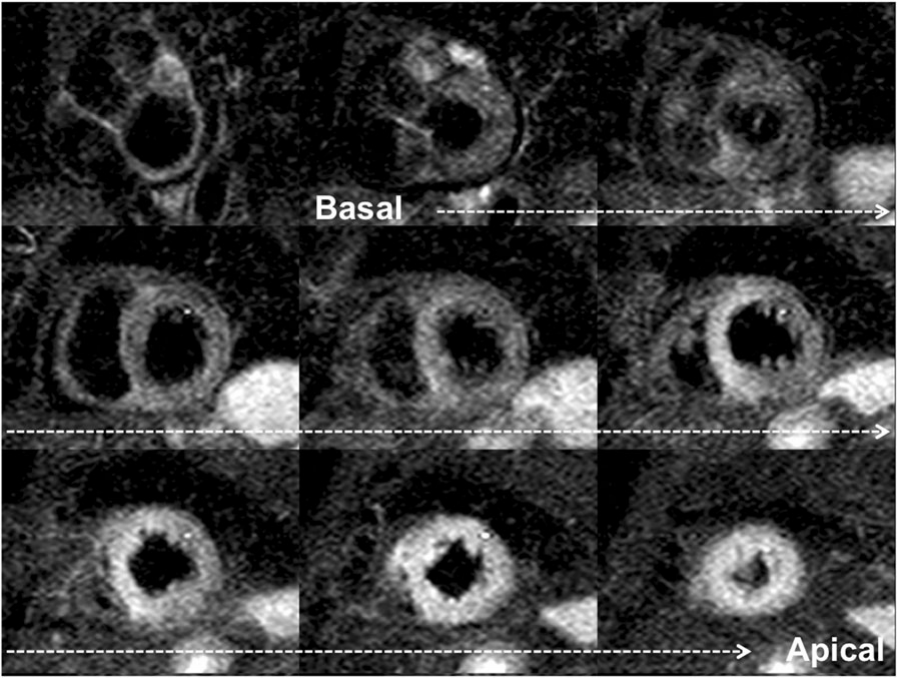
Myocardial edema. T2-weighted triple-inversion recovery short-axis view showing transmural signal hyperintensity in the mid-apical segments of left ventricle
